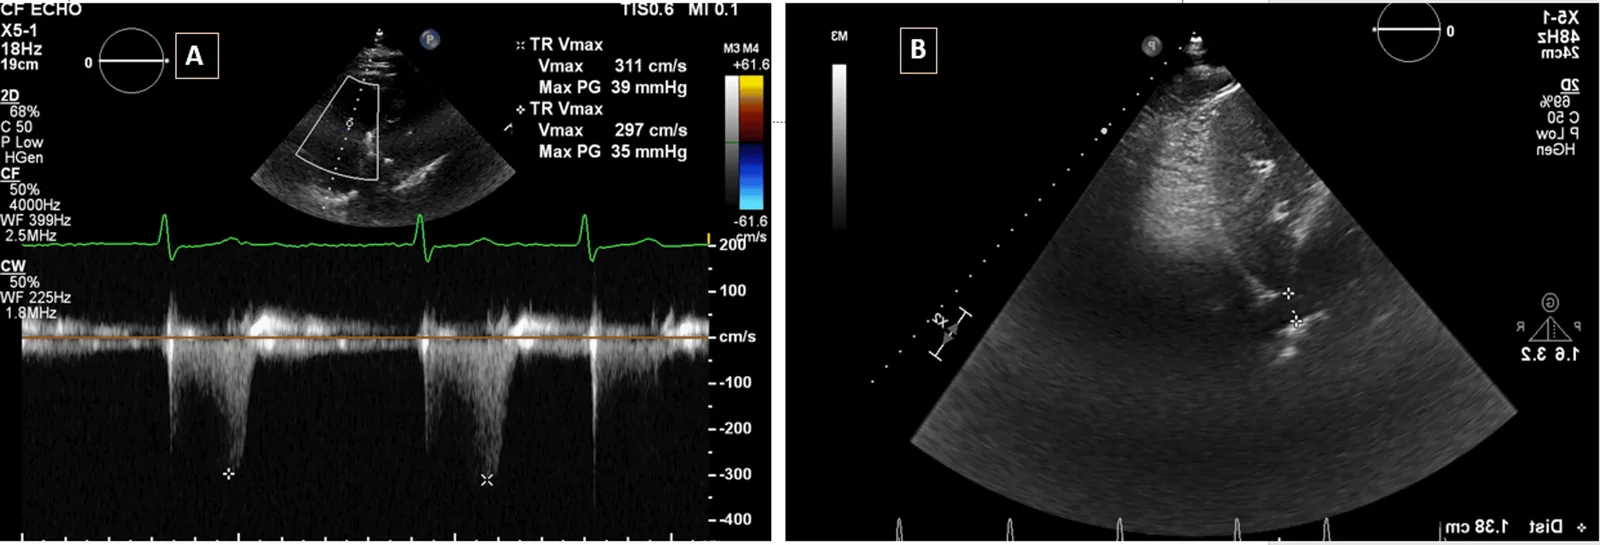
Echocardiographic estimation of mildly elevated right ventricular systolic pressure at initial presentation (A) Continuous-wave Doppler of the tricuspid regurgitation jet used for estimation of right ventricular systolic pressure (RVSP). The formal echocardiographic interpretation reported a peak tricuspid regurgitation velocity of 3.02 m/s with an assumed right atrial pressure of 10 mmHg, yielding an estimated right ventricular systolic pressure of 46.6 mmHg. (B) Subcostal view demonstrating the inferior vena cava (diameter 1.38 cm), which was used in conjunction with respiratory collapsibility for estimation of right atrial pressure.
